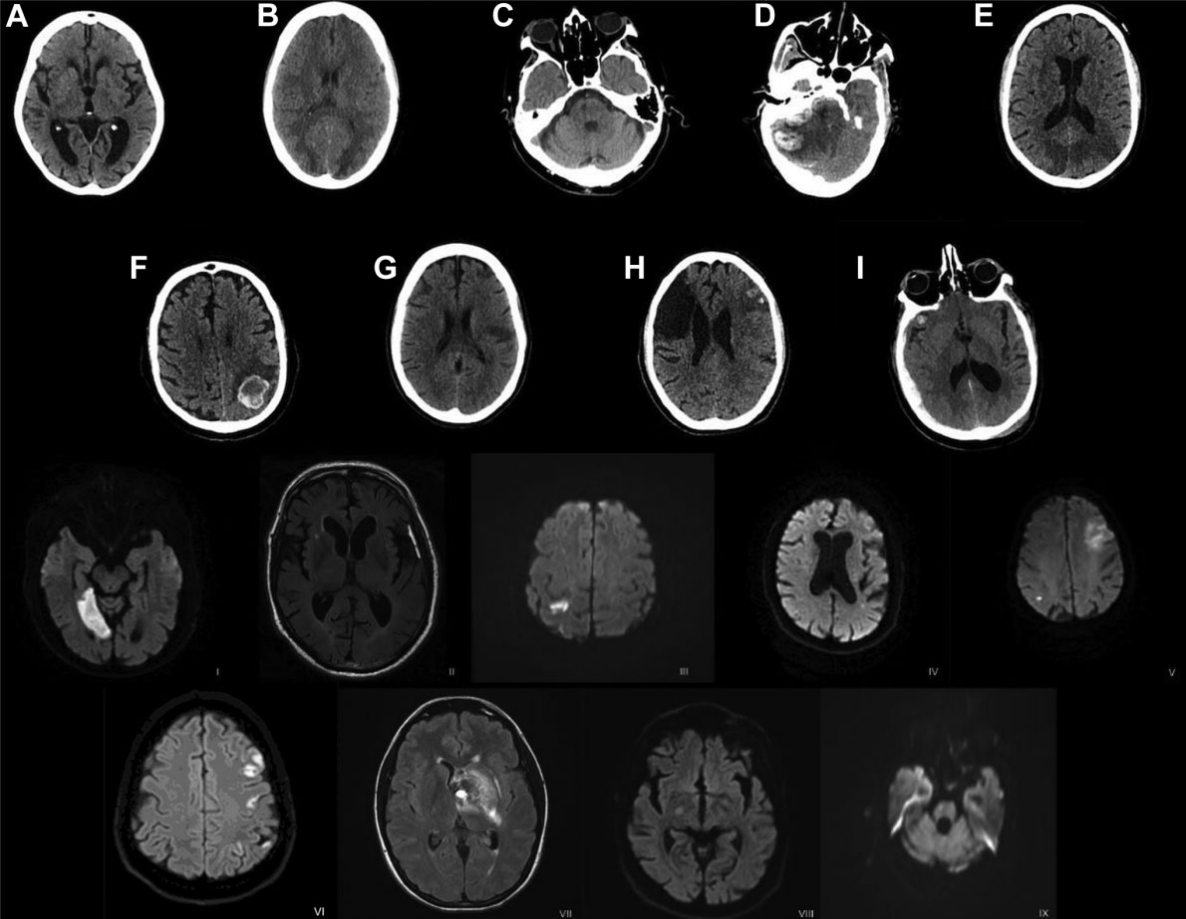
Most representative image from each of the 18 patients with SARS-CoV-2 and acute or subacute cerebrovascular events. A-I are representative CT images; I-IX are representative MRI images. (A) Subacute lacunar infarct within left basal ganglia; (B) Bi-occipital multifocal subcortical white matter hypoattenuation with gray-white junction blurring; (C) Subacute/chronic lacunar infarct in the right paramedian pons; (D) Acute large right cerebellar intraparenchymal hemorrhage with associated mass effect; (E) Acute infarcts involving the right PCA territory and left PCA/MCA border zone; (F) Multifocal intraparenchymal hemorrhage; (G) Acute/subacute infarct in the left posterior frontal lobe; (H) Acute left frontotemporal infarct with asymptomatic hemorrhagic conversion. Old right frontoparietal infarct with encephalomalacia; (I) Acute subdural hematoma overlying right lateral cerebral hemisphere with mass effect and intraparenchymal hemorrhage. (I) Acute right medial occipital infarct; (II) Thin hyperintense subdural hematoma involving the left frontotemporal region; (III) Mult-focal acute/subacute infarct within the right frontoparietal lobes; (IV) Acute left frontoparietal lacunar infarct; (V) Acute infarcts of the bilateral cerebral hemispheres; (VI) Multi-focal acute/subacute cortical infarcts in the left MCA territory (VII) Acute hemorrhagic cavernous malformation with associated developmental venous anomaly centered in the left hypothalamic region; (VIII) Small acute/subacute infarct in the posterior limb of the right internal capsule; (IX) Subacute infarct in right midbrain. (SARS-CoV-2 = severe acute respiratory syndrome coronavirus 2, CT = computed tomography, MRI = magnetic resonance imaging, PCA = posterior cerebral artery, MCA = middle cerebral artery).
